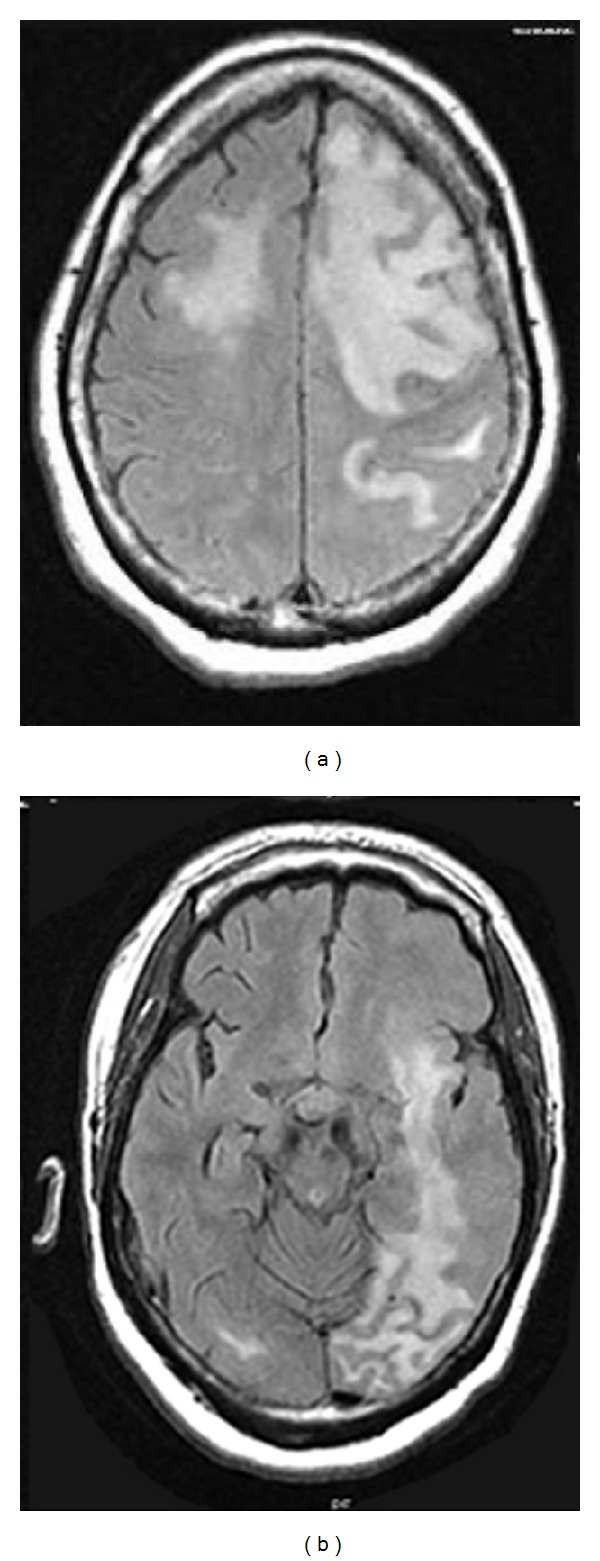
MRI brain-diffuse increased FLAIR signal throughout the left cerebral hemisphere.
